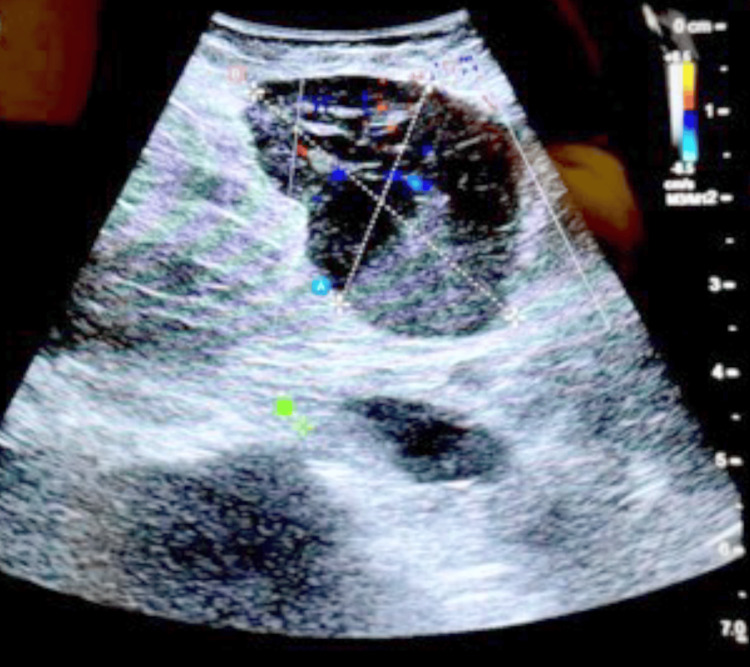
Intraoperative real-time ultrasonography (BK5000; BK Medical) during RALA in a patient with suspected adrenal metastasis from hepatocellular carcinoma. Ultrasonography delineated lesion margins and adjacent vascular structures, improving localization and guiding precise dissection. Representative single-patient image (no statistical comparison performed). RALA, robotic-assisted laparoscopic adrenalectomy
